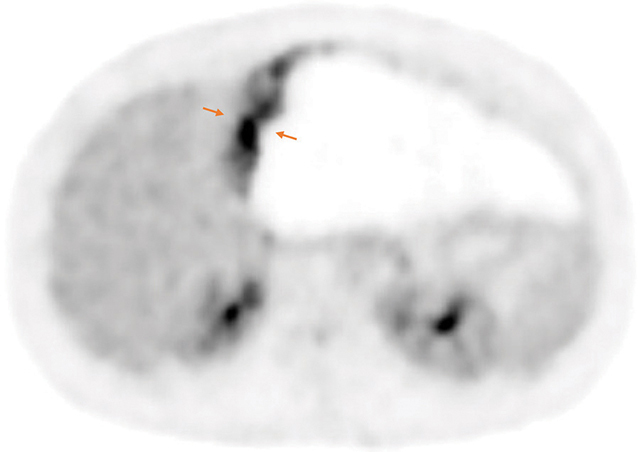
Axial 18FDG PET-CT. High 18FDG uptake in the left lesion part (orange arrow).
